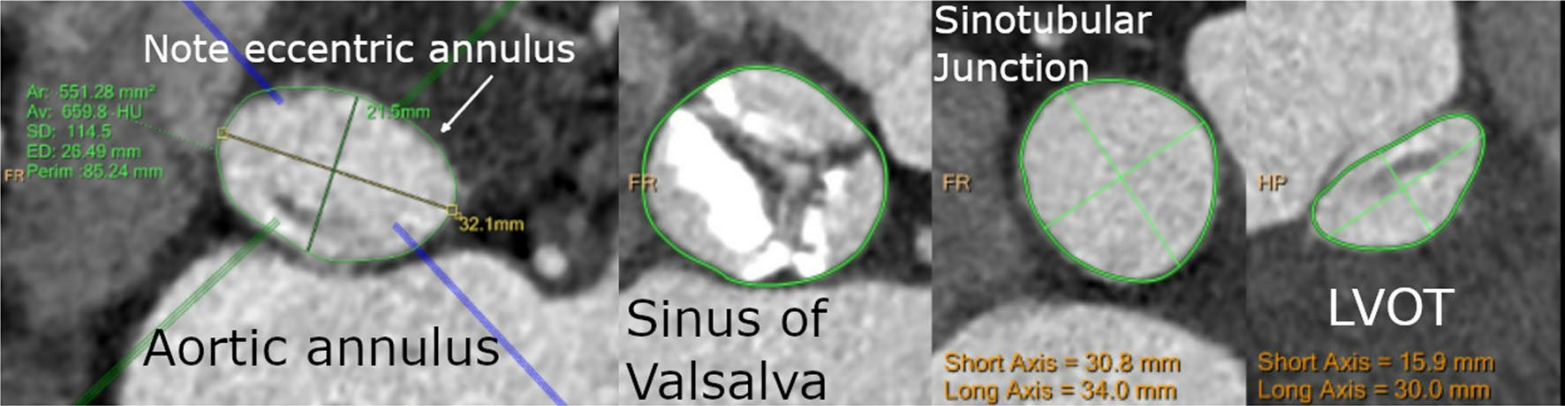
Patient 1 pre-Op CT scan
